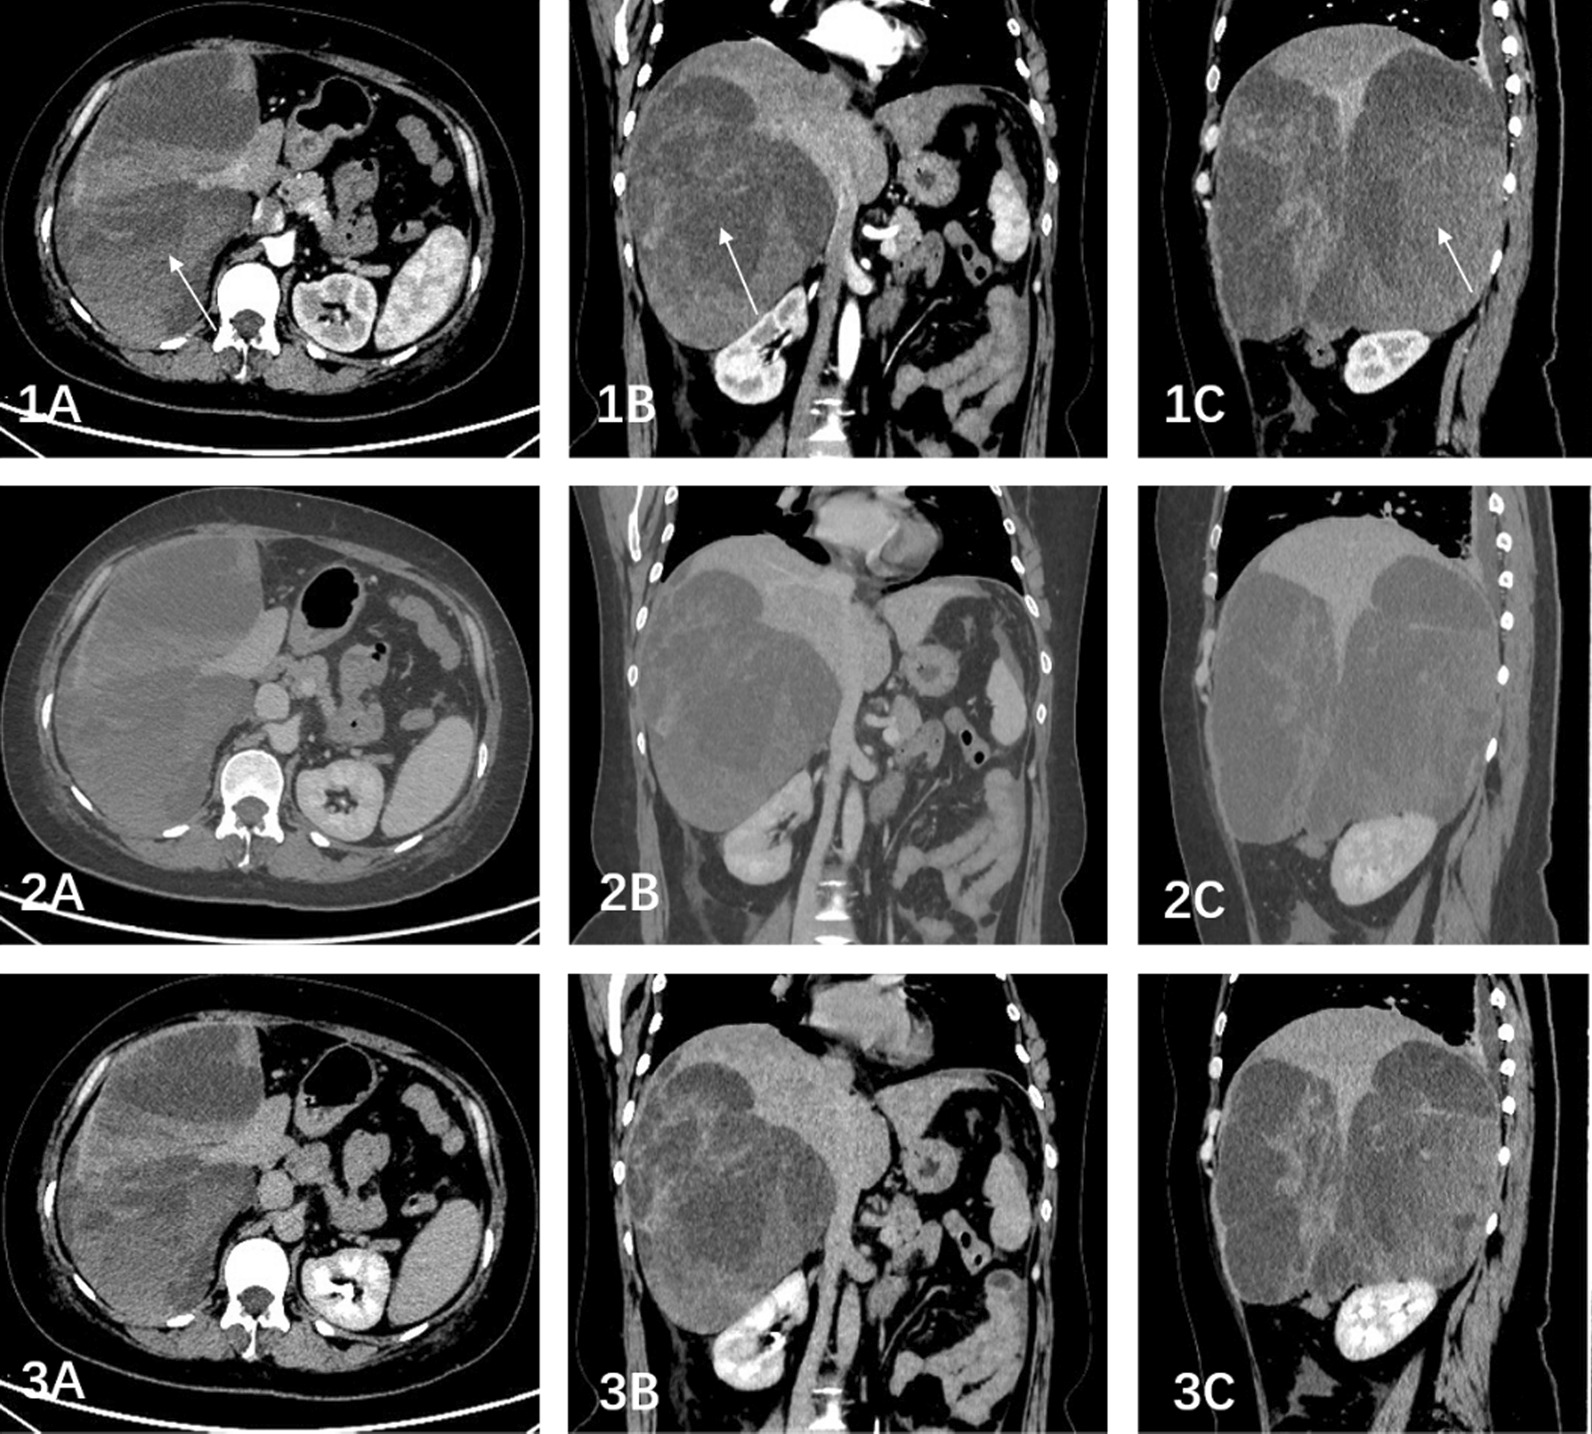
(2019-06-24) Abdominal enhanced-CT: multiple heterogeneous dense masses with regular morphology and clear boundaries between the right lobe of liver and the diaphragm. The largest one was about 13.4 × 9.2 cm and the CT value was between 20-45Hu with no obvious enhancement on enhanced scans. (1A: Axial arterial phase; 1B: Coronary arterial phase; 1C: Sagittal arterial phase; 2A: Axial portal phase; 2B: Coronary portal phase; 2C: Sagittal portal phase; 3A: Axial delayed phase; 3B: Coronary delayed phase; 3C: Sagittal delayed phase.)
